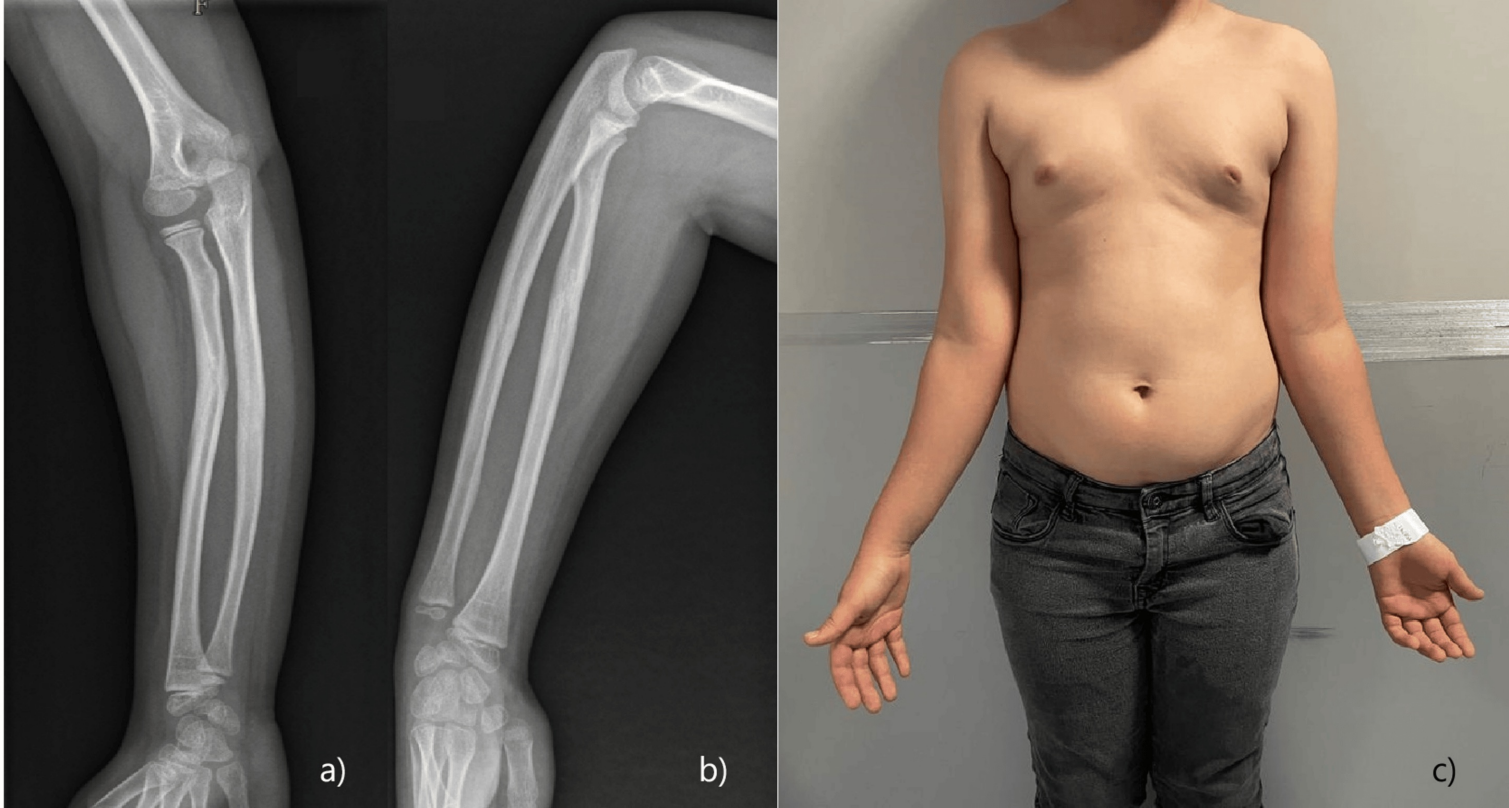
Re-evaluation at nine months after the injury a) Anteroposterior view of forearm radiograph; b) side view of forearm radiograph; c) physical examination with right arm rotational deformity and supination limitation.
